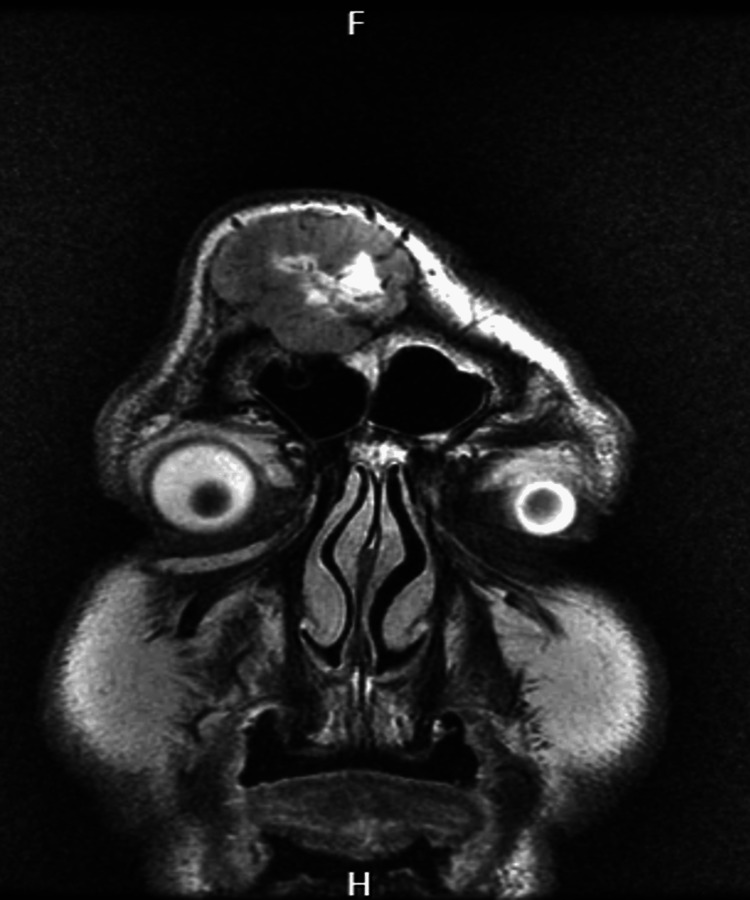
Cranioencephalic MRI in coronal section The image shows a coronal section in T2.
